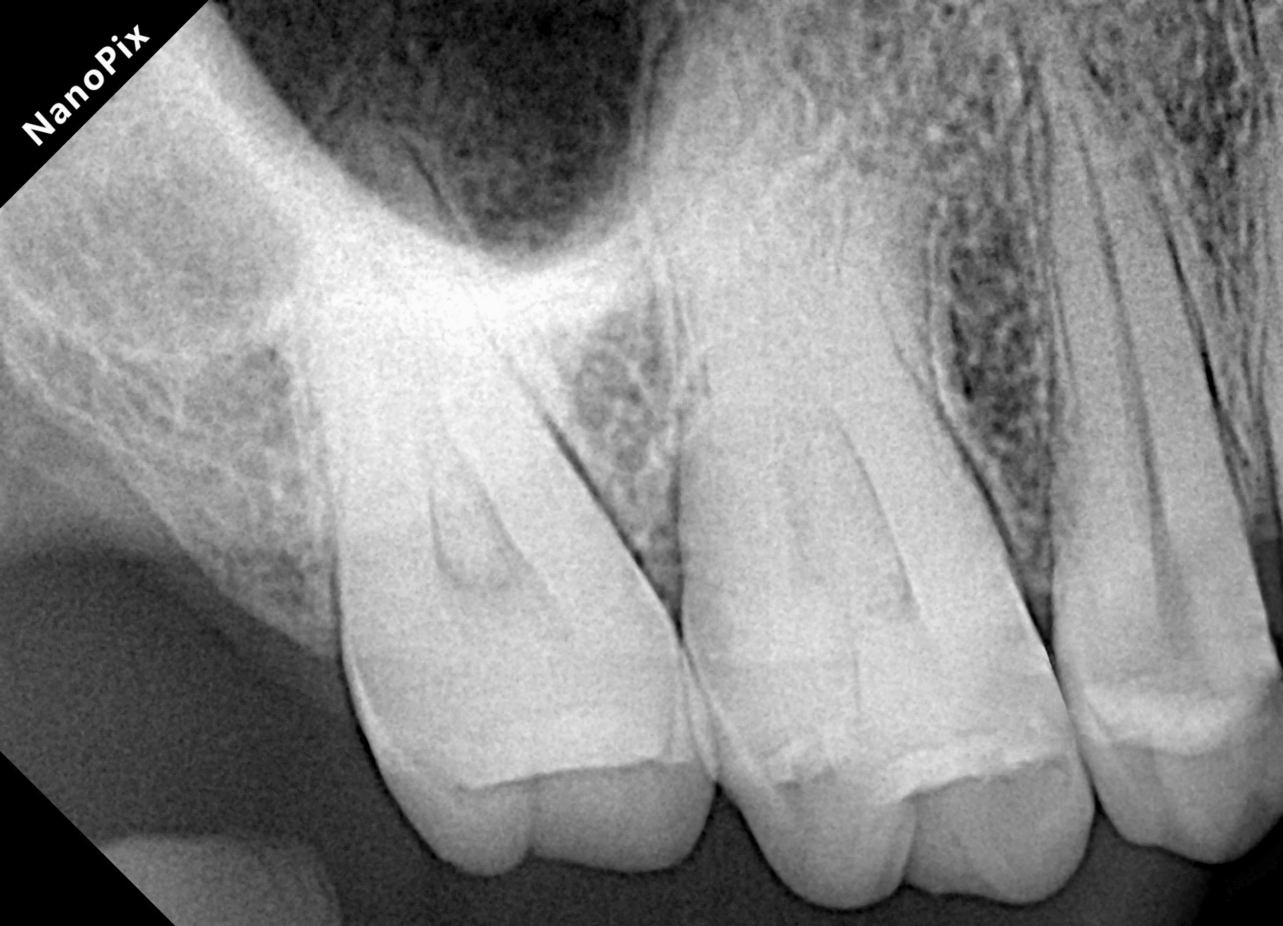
Intraoral periapical radiograph (IOPA) of tooth #16.
